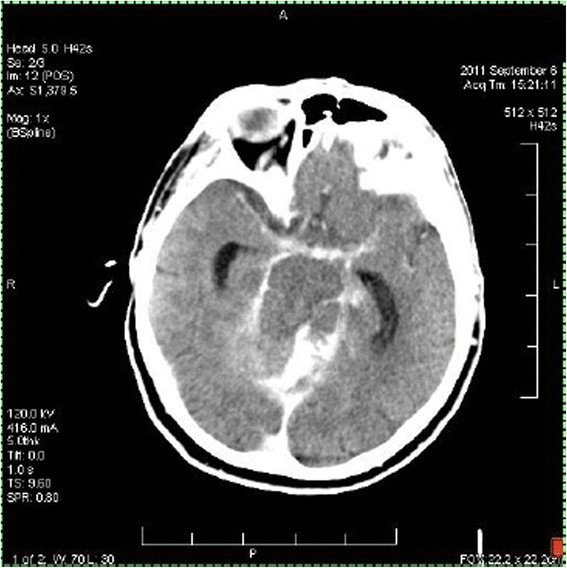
Noncontrast head computed tomogram obtained once the patient arrived in the intensive care unit.
